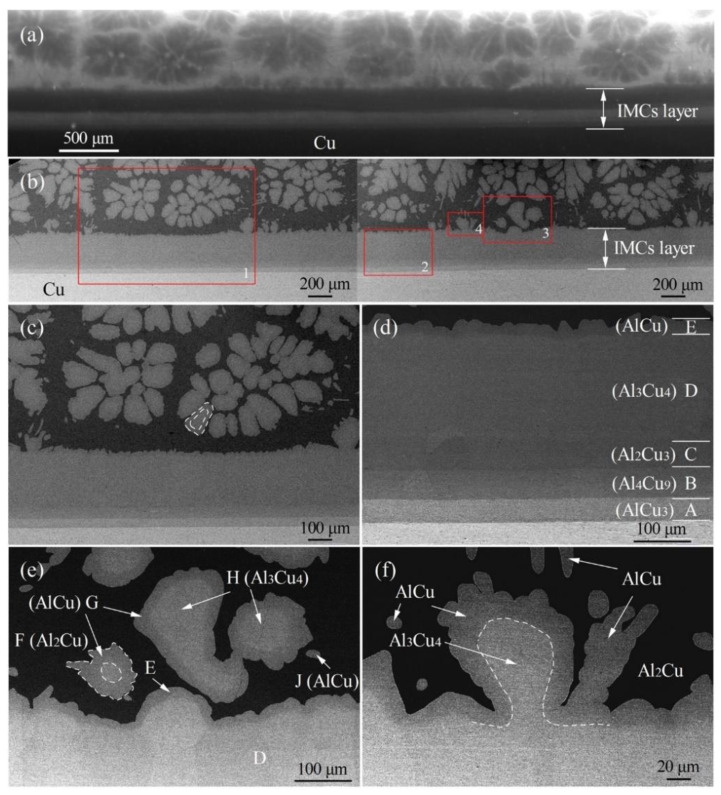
Characterization of interfacial microstructure in Al/Cu sample: (a) in situ radiograph, (b) SEM image corresponding to Figure 5a, (c–f) enlarged images of red rectangular areas in Figure 5b.
